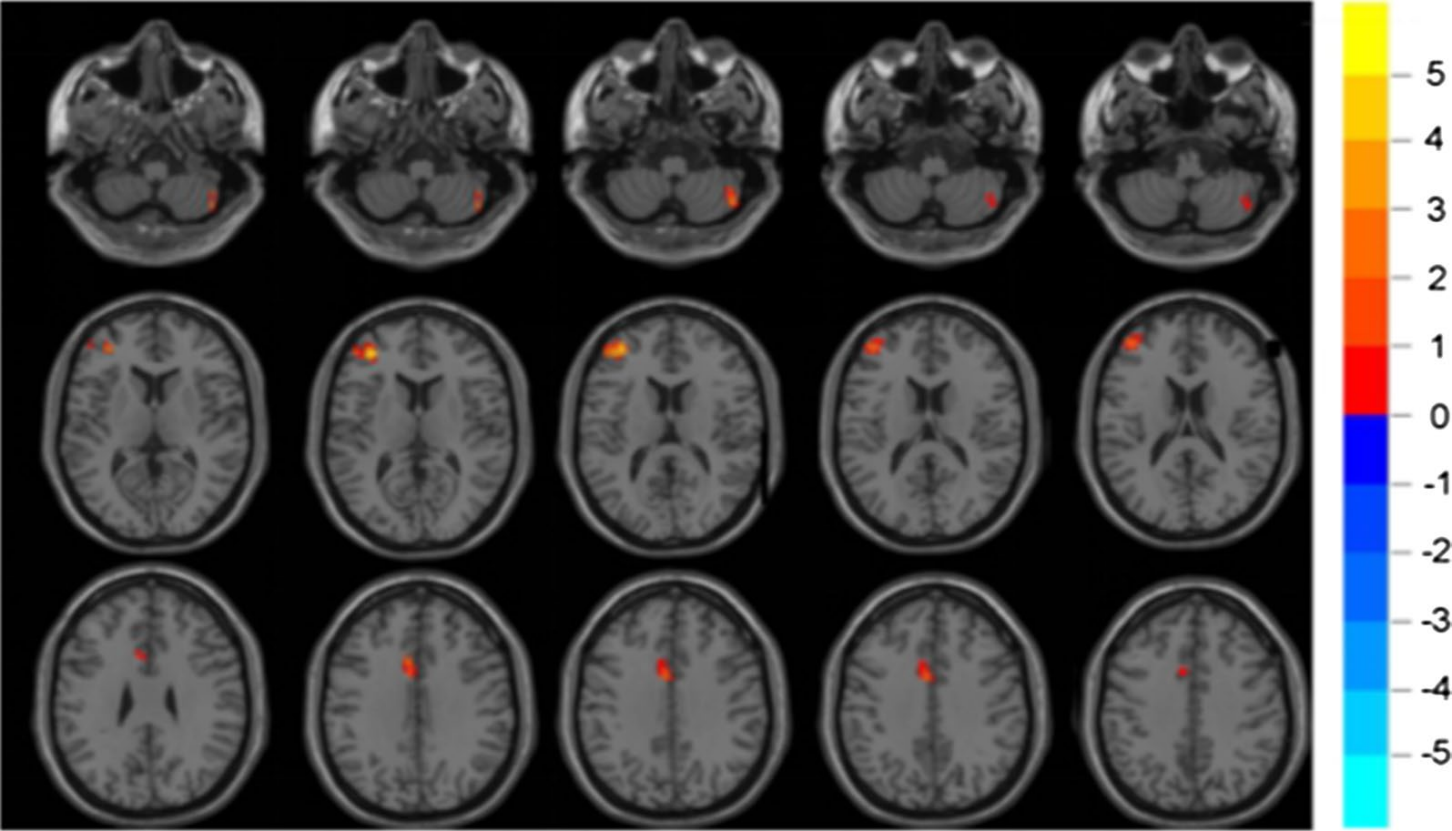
Statistically significant differences between the perimenopausal and control subjects are shown in a ReHo map of the whole-brain with MRI. The perimenopausal subjects showed a significant ReHo increase in the right posterior cerebellum (region 2), left middle frontal gyrus and left middle cingulate gyrus.A T-score bar is shown on the right. Red and green denote increases and decreases in ReHo, respectively
